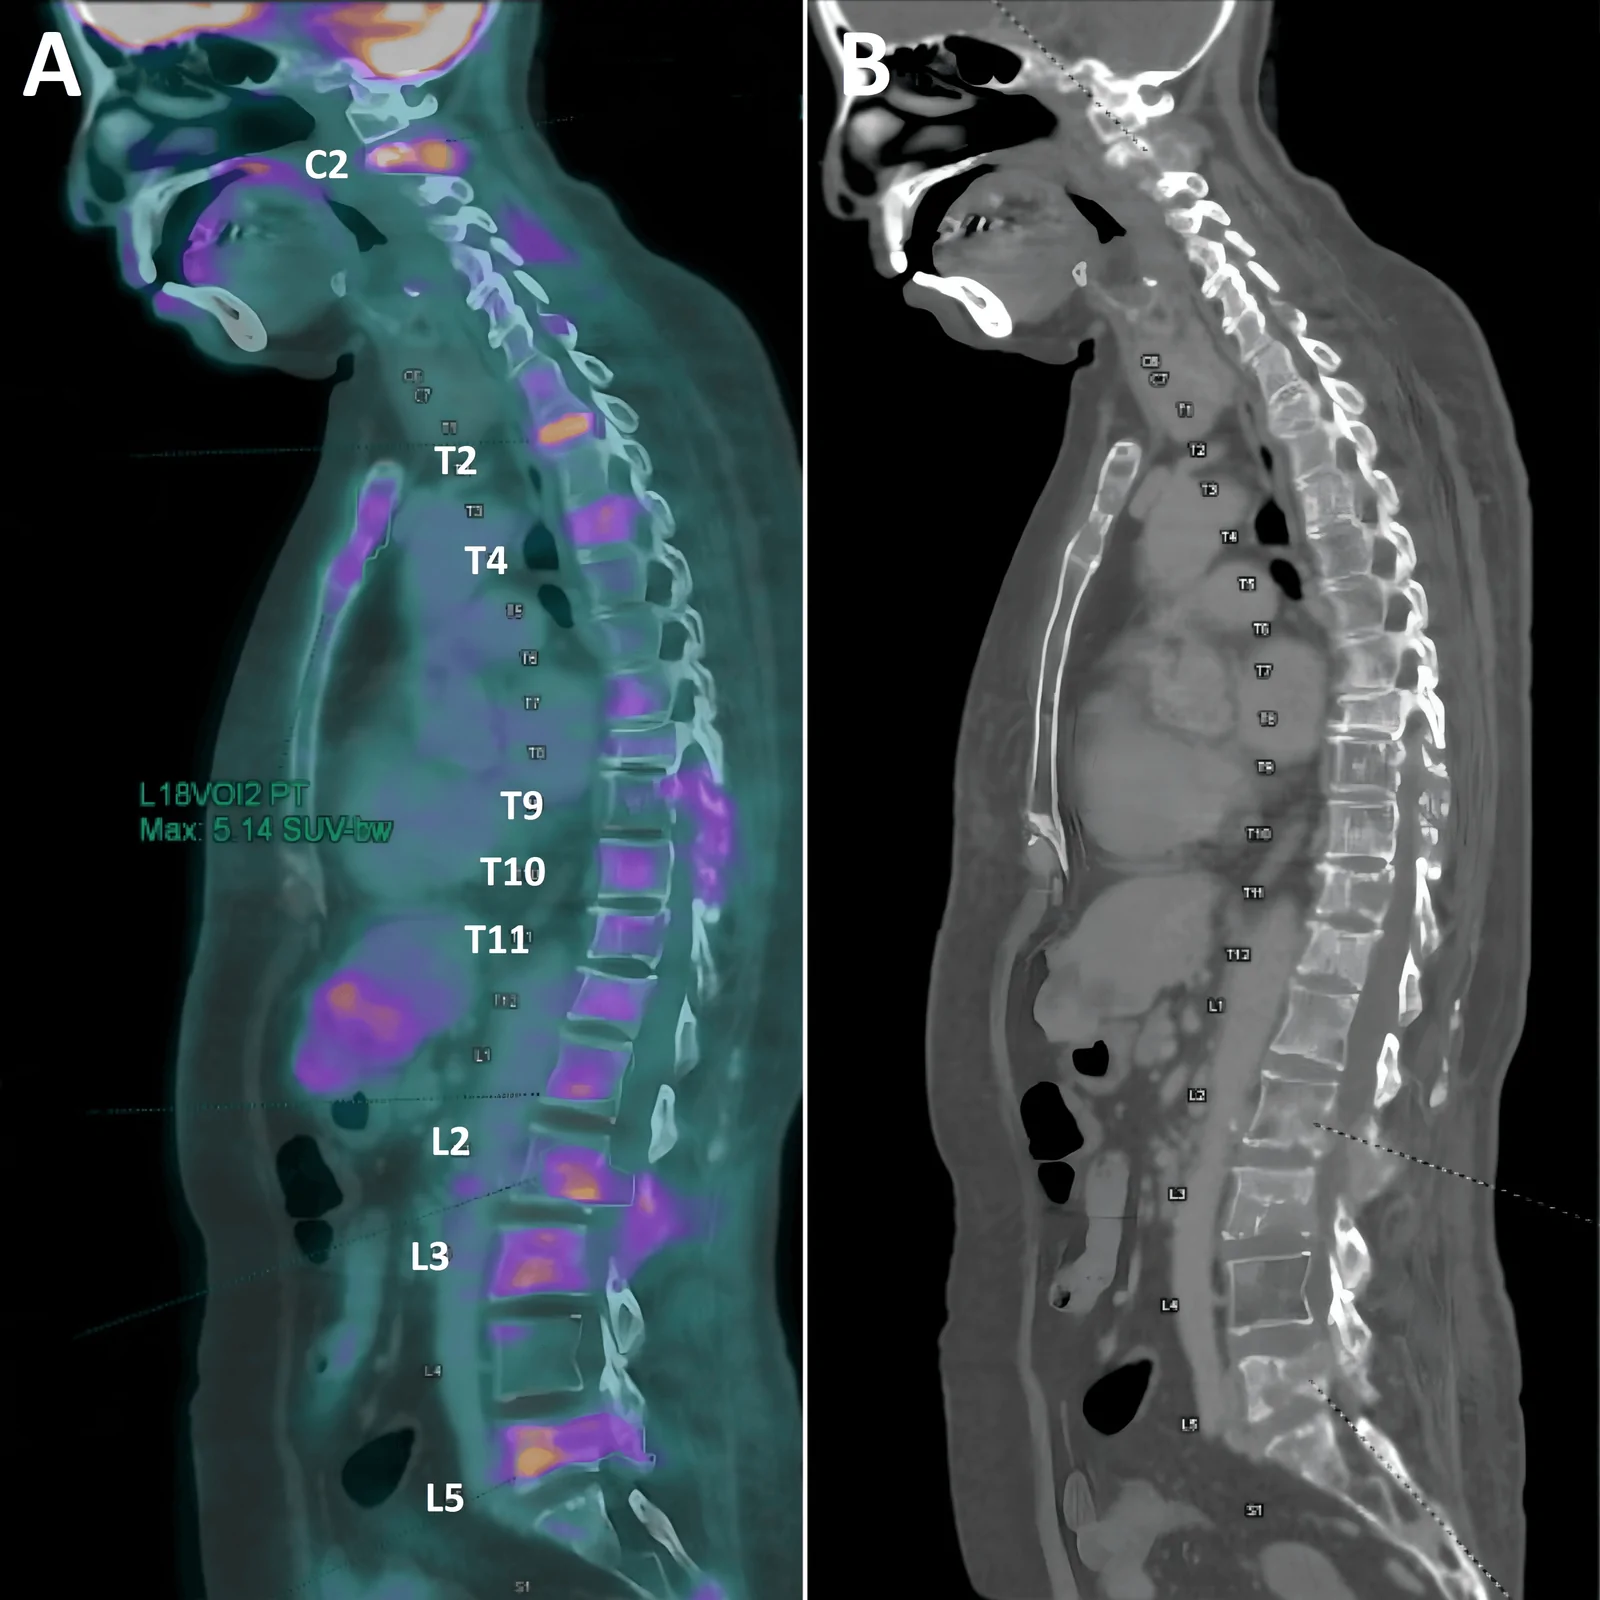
PET-CT images demonstrating multiple new and progressive skeletal metastases 26 months after initial diagnosis A) Coronal fused PET-CT image shows widespread hypermetabolic osseous lesions involving the vertebral column (C2, T2, T4, T9–T11, L2, L3, L5), pelvis, sternum, bilateral scapulae, bilateral ribs, both humeri, and left femur. Note intraspinal extension at multiple levels (e.g., C2, T9–T11). (B) The corresponding coronal CT image better delineates the osseous destructive changes and anatomical extent of the metastases.
